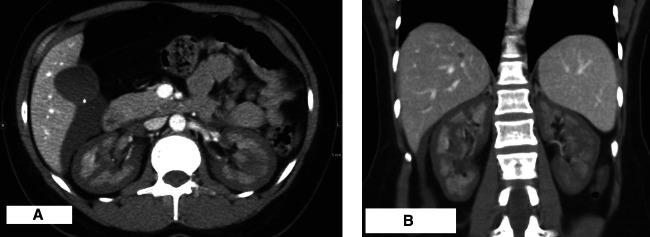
Reverse rim sign in renal cortical necrosis. (A) Axial contrast-enhanced CT image demonstrates symmetric cortical hypoenhancement with preserved medullary enhancement of the reverse rim sign. There is a small amount of fluid in the hepatorenal recess. (B) Coronal contrast-enhanced CT image demonstrates a similar finding of symmetric cortical renal hypoenhancement with preserved medullary enhancement of the reverse rim sign. CT, computed tomography.
